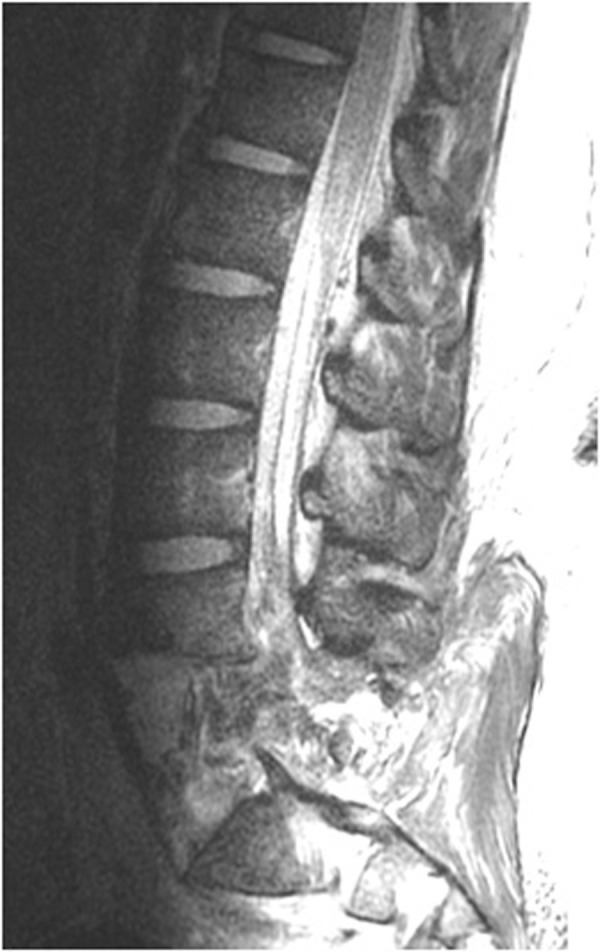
Preoperative parasagittal MRI This is a preoperative parasagittal MRI showing gross ligamentous instability and complete obliteration of the thecal sac at the level of injury.
